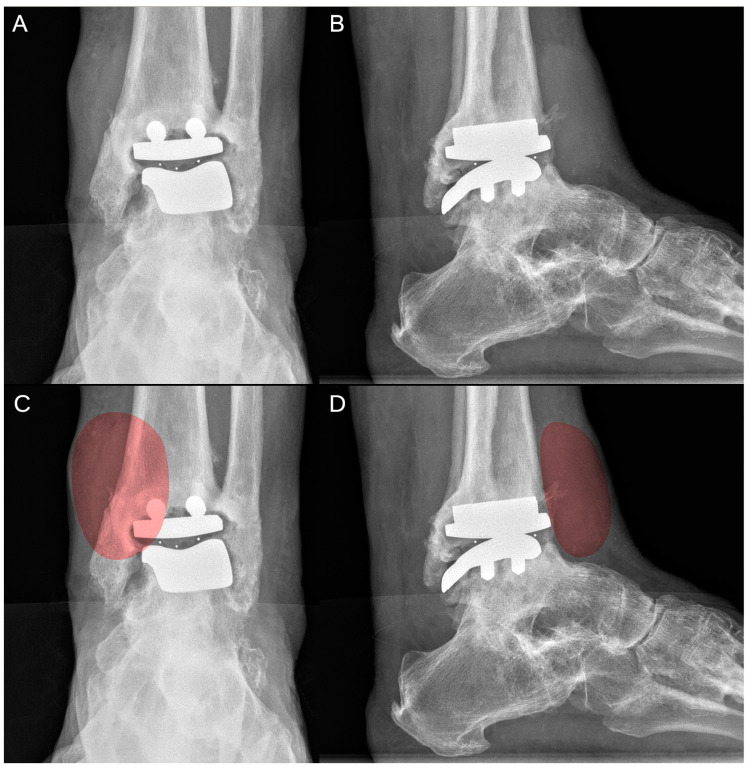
Radiographic evaluation revealed mild resorption at the prosthesis–bone interface, accompanied by signs of tibial and talar component loosening and alterations in the physiological bone contour (A). A periarticular mass extended medially and anteriorly from the tibial component (B). The mass was identified, and it is highlighted in red in both the anteroposterior (C) and lateral (D) views.
